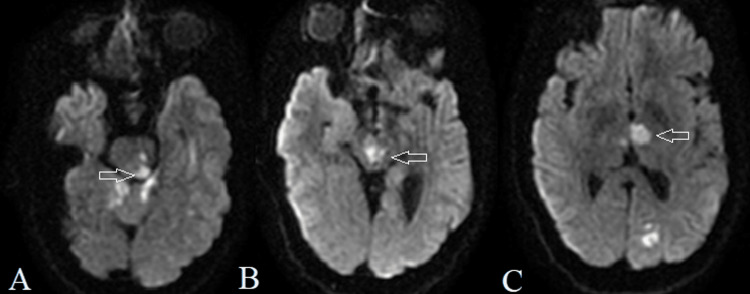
Cranial magnetic resonance DWI showing hyperintense signal at pontine tegmentum extending to the midbrain (A and B), thalami, and left occipital lobe (C) (arrows). DWI: diffusion-weighted imaging
